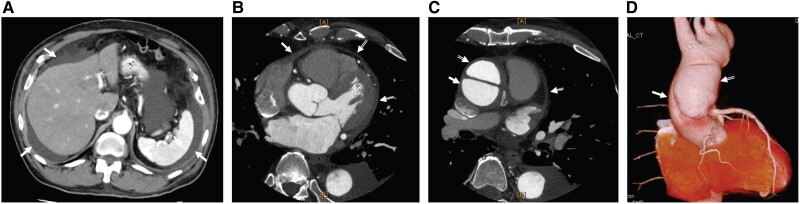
Pre-operative CT. (*A*) The arrows indicate ascites. (*B*) The pericardium around the ventricles is thickened, but the degree of thickening differs in each area: single, double, and triple arrows. No pericardial effusion is observed, and both the left and right ventricles are smaller than the left and right atria. RA, right atrium; LA, left atrium; RV, right ventricle; LV, left ventricle; V, sinus of Valsalva. (*C*) The ascending aorta is dissected; the double arrows indicate the false lumen, and the single arrow indicates the true lumen. The triple arrows indicate thickened pericardium. (*D*) Three-dimensional CT also shows a false lumen, indicated by double arrows, diagnosed as DeBakey type II aortic dissection.
